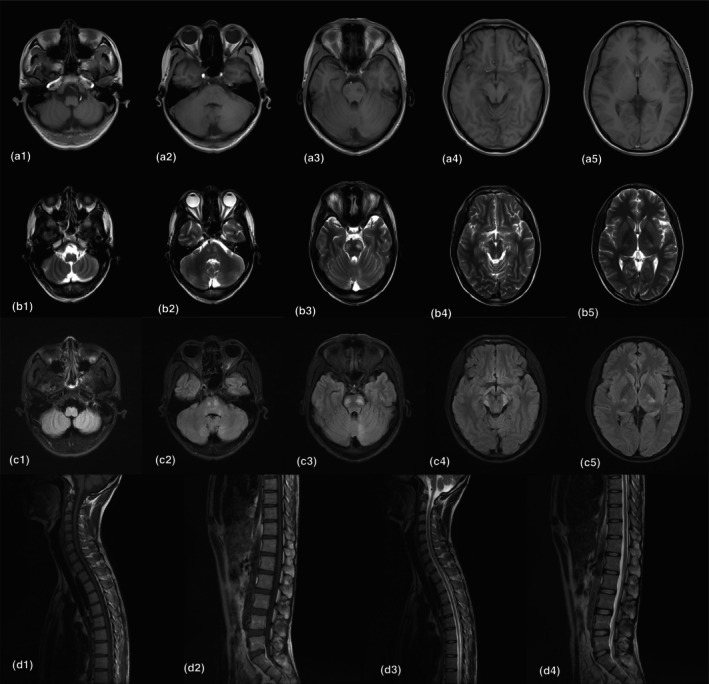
Magnetic resonance imaging (MRI). (a–c) Axial brain MRI scans showed distribution of lesions at five levels: cerebellum, cerebellopontine angle, brainstem, midbrain, and internal capsule. a = T1‐weighted MRI, b = T2‐weighted MRI, c = fluid‐attenuated inversion recovery. (d) Saggital spinal cord MRI scans showed thoracic spinal cord atrophy.
